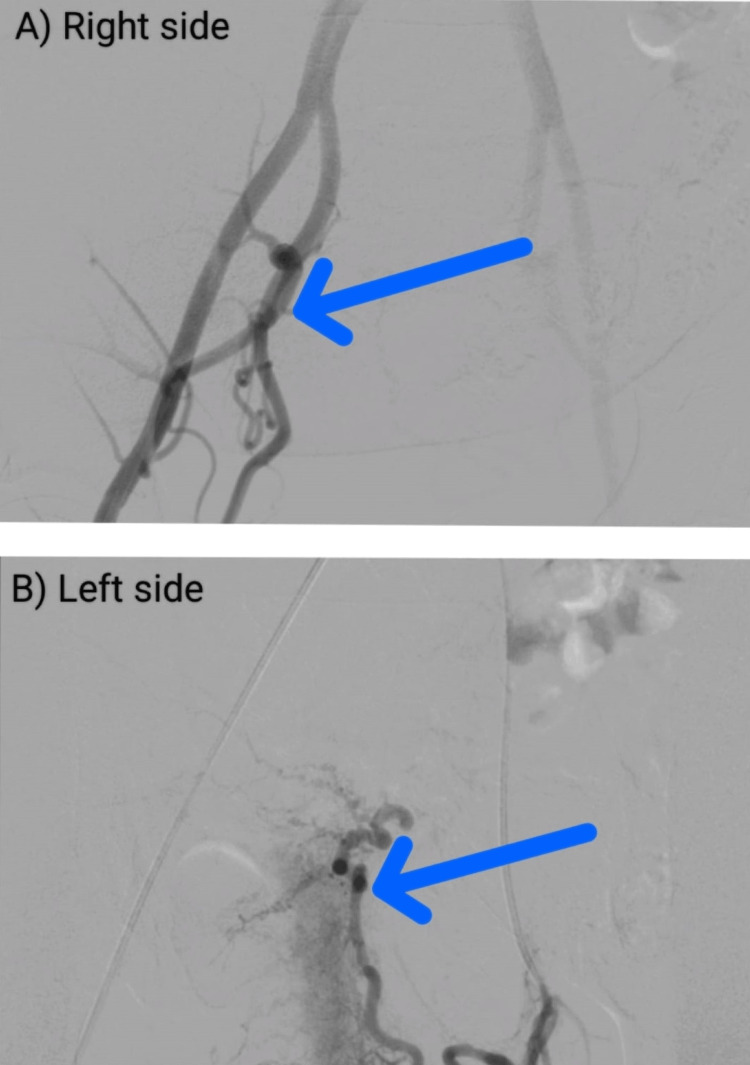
An X-ray image of the angiogram done after the uterine artery embolization: A) right side and B) left side
